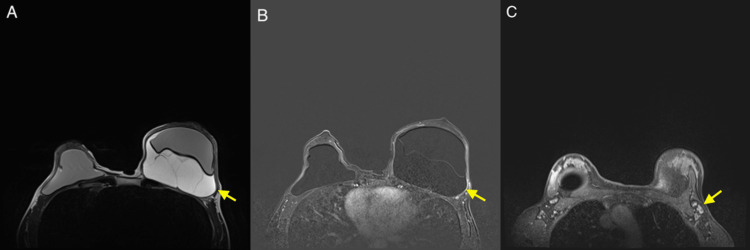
(A) Axial T2-weighted MRI of the breasts demonstrating a complex T2 hyperintense fluid collection with internal septations surrounding the left breast pre-pectoral silicone implant, displacing the implant anteriorly. (B) Axial T1 post-contrast with fat saturation image demonstrating only minimal peripheral enhancement along the lateral aspect of the peri-implant collection capsule wall. (C) Axial T1 post-contrast with fat saturation image demonstrating asymmetrically prominent left axillary lymph node with associated cortical thickening.
